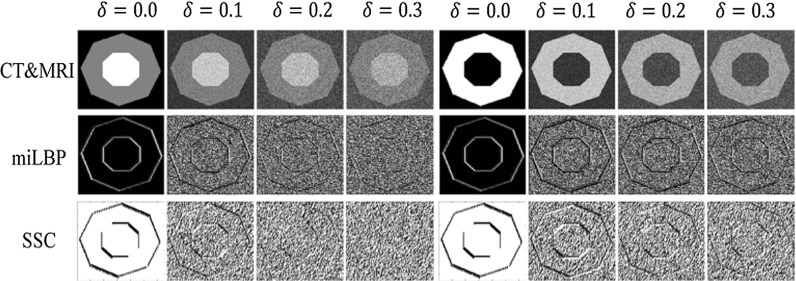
Artificial images representing CT and MRI with different levels of noise and their corresponding descriptor images of miLBP and SSC. The *left four* images are the CT images. For 2D SSC, we only show the first-dimensional values of the 4D vector-valued descriptor
